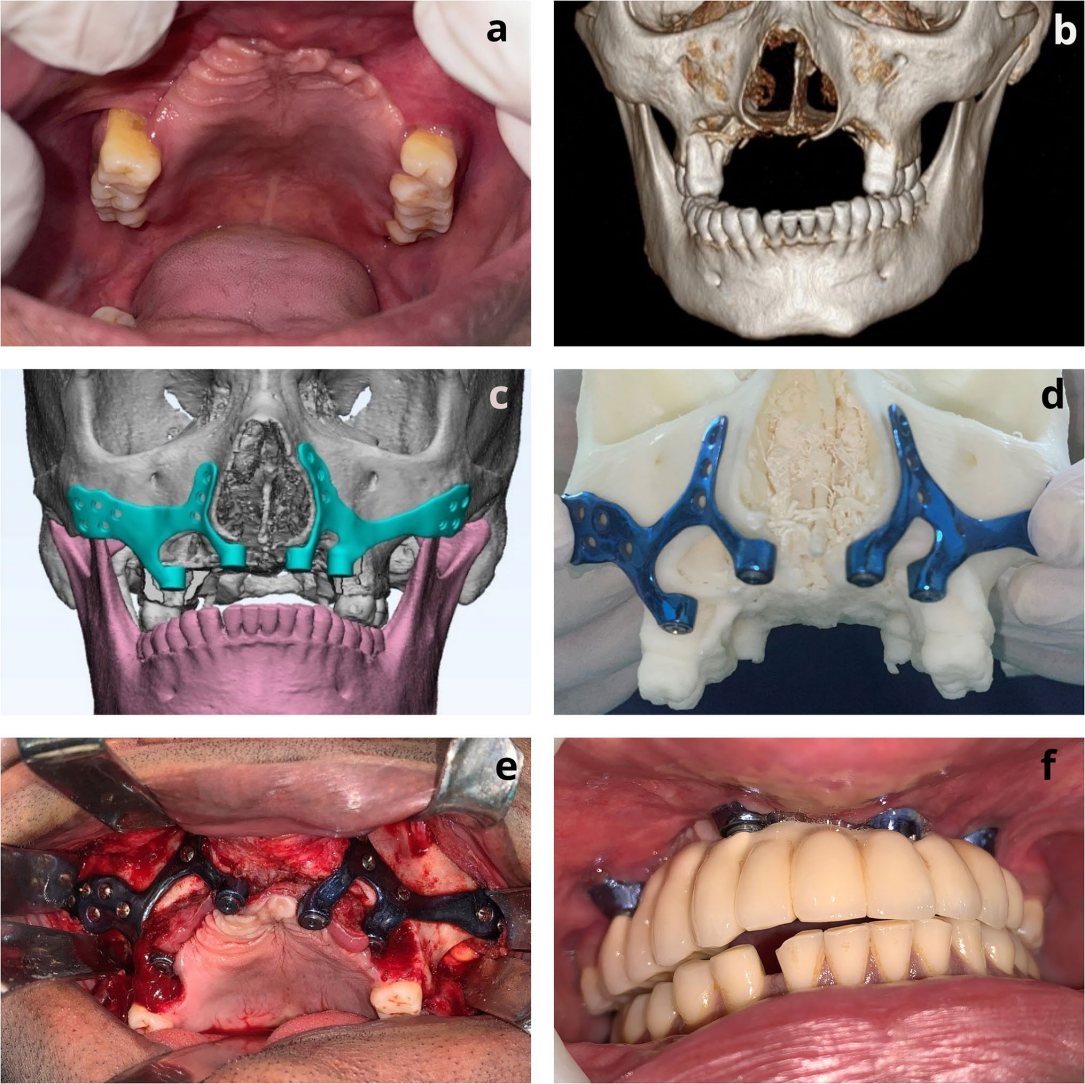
Rehabilitation of MRONJ defect (Brown Type IIc); a Intra-oral view of patient’s edentulous anterior maxillary arch resulting due to MRONJ treatment; b 3D CT view depicting the paucity of bone in the anterior dentoalveolar region; c VSP for designing of framework for rehabilitation of the defect; d Fabricated framework seated on the STL model; e The framework placement and fixation intra-operatively (depicts the modification of the incision to leave a cuff of crestal tissue to avoid iatrogenic oro-antral communication during surgical exposure).; f Intra-oral view of occlusion after fixation of the prosthesis to the framework
